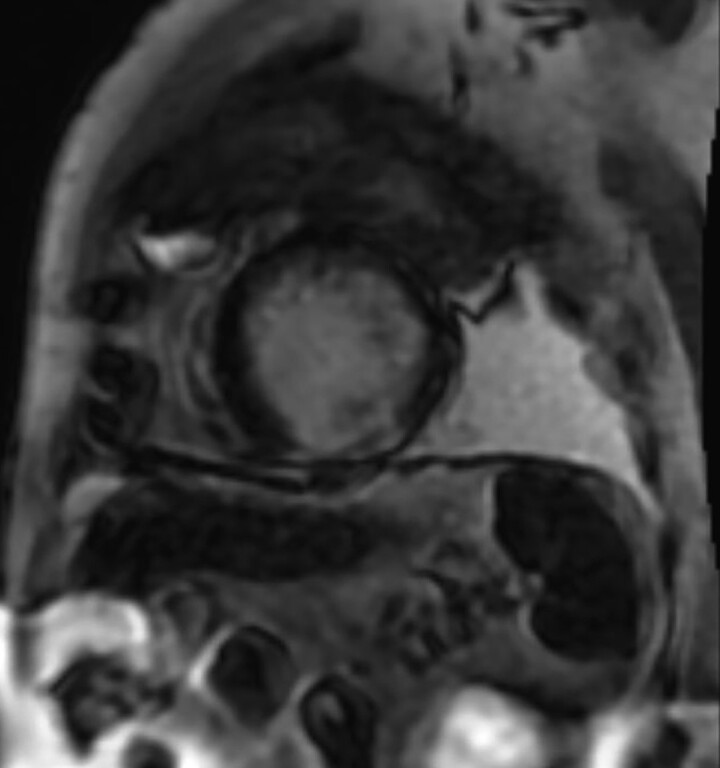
Cardiac magnetic resonance imaging. Short axis late-gadolinium enhancement imaging showing scar in the inferno-lateral wall.
